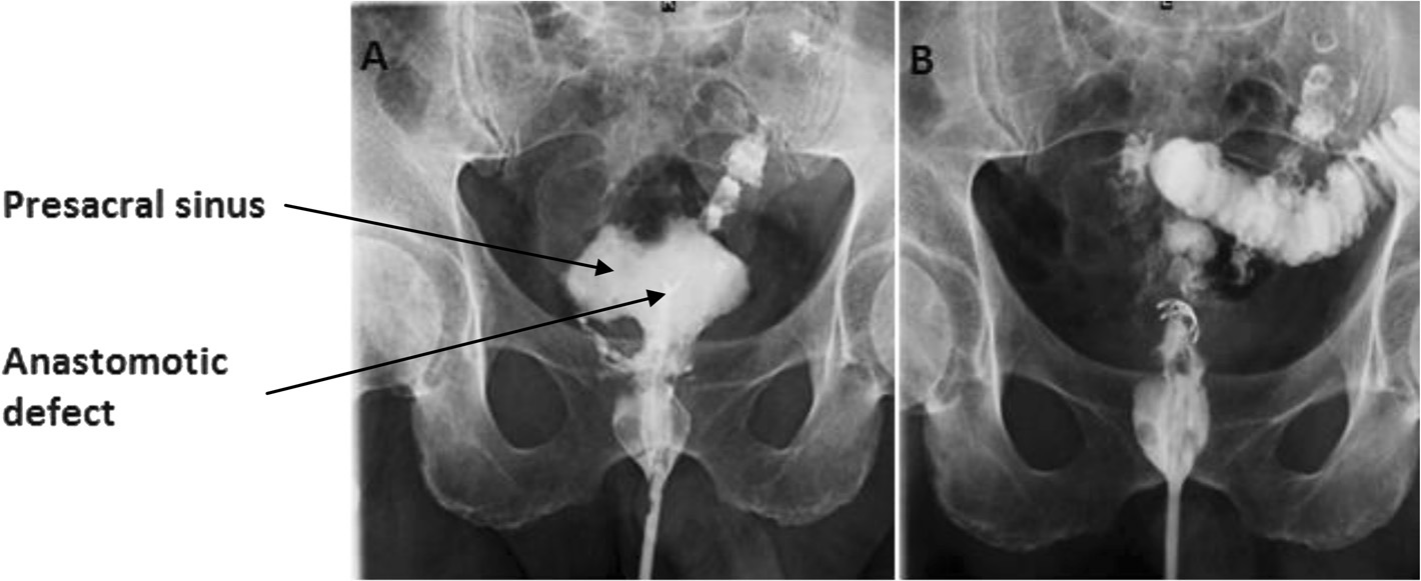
Contrast radiography showing a cave of 10 × 9 × 4 cm and a defect of about 1.5 cm before intervention (a, *arrows*) and after applying the OTSC (b)
